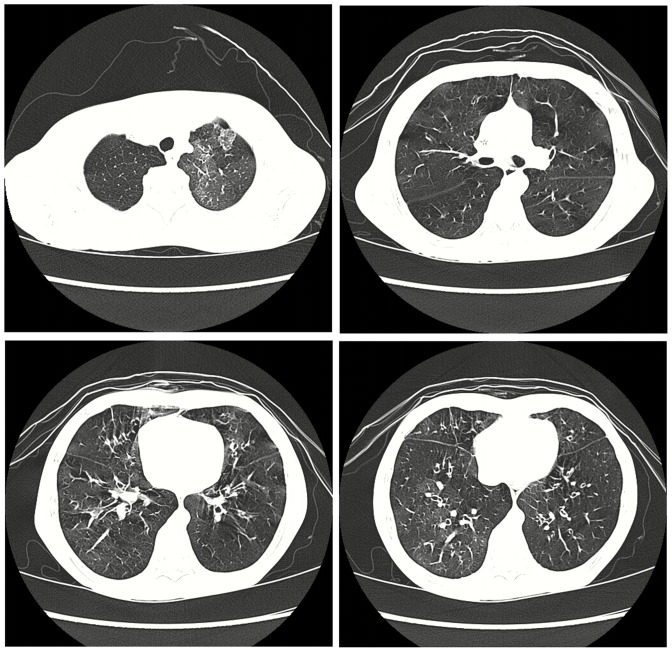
HRCT of patient No.-glass pattern with air trapping and bronchial thickening.
